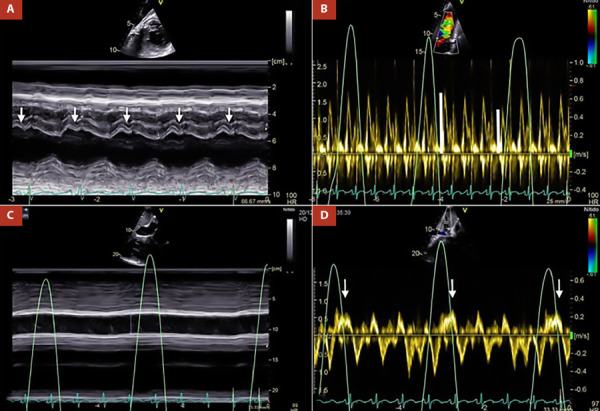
A. M mode of interventricular septum. Pericardial notch is evident (white arrows), B. Pulsed Doppler at the level of the tricuspid valve. There is transtricuspid variability of 25% (white lines), C. M mode of the inferior vena cava, which is markedly dilated without respiratory variability, D. Pulsed Doppler of the suprahepatic vein, reverse diastolic flow is shown in expiration (white arrows).
